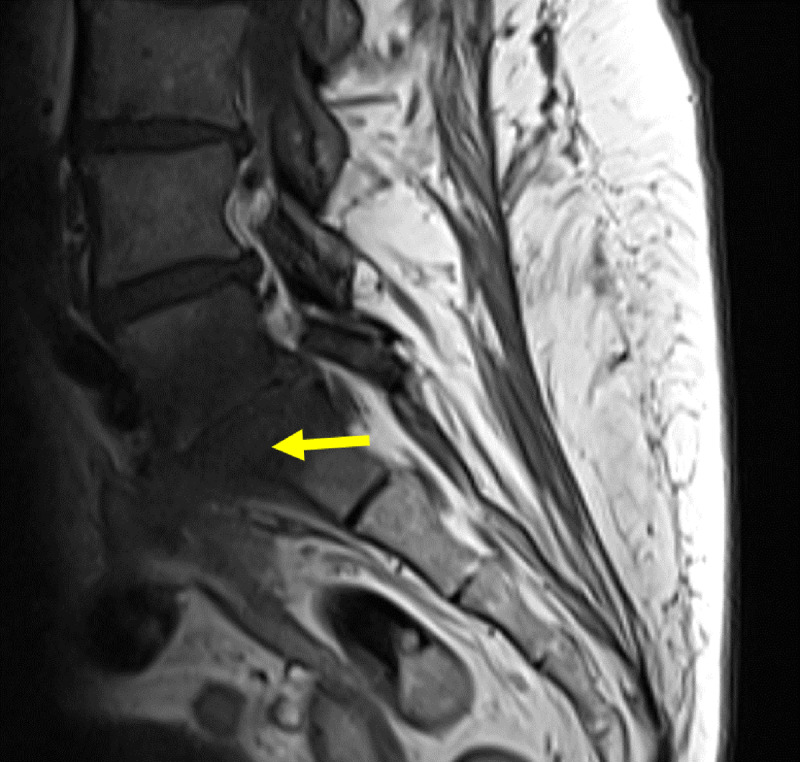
Sagittal contrast MRI in T1 weighting: A medullar bone oedema is seen on either side of the L5-S1 disk. Small collections surround L5-S1 with the largest being 20mm in diameter located on the left psoas muscle with a wall that was intensified by the contrast. This abscess extends posteriorly to the anterior peridural space, the lumbar vertebral bodies, and the last thoracic vertebra.
